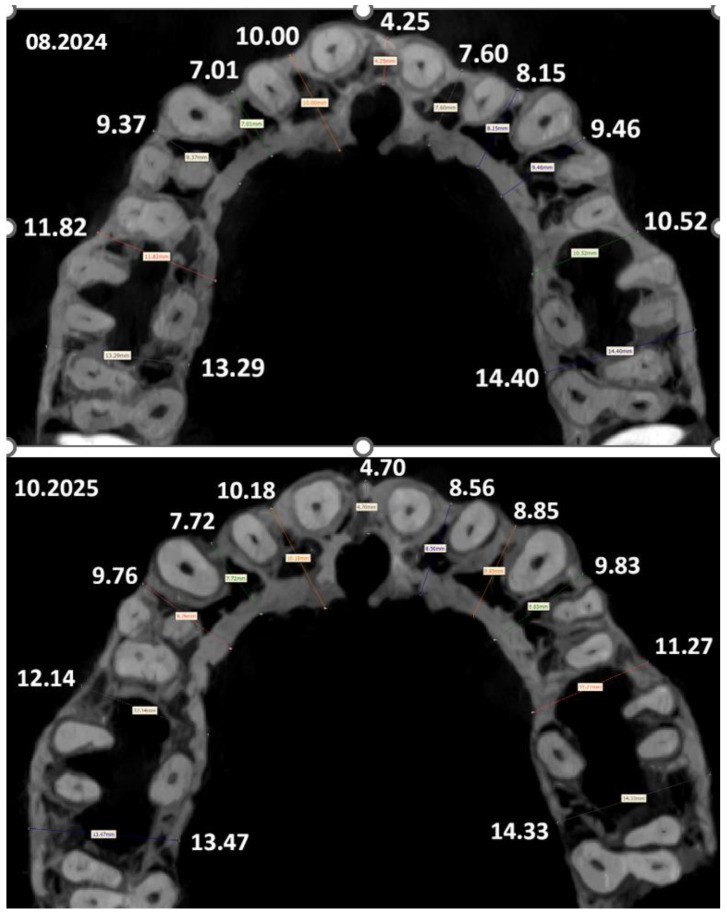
Maxillary alveolar ridge width before and after treatment (CBCT).
